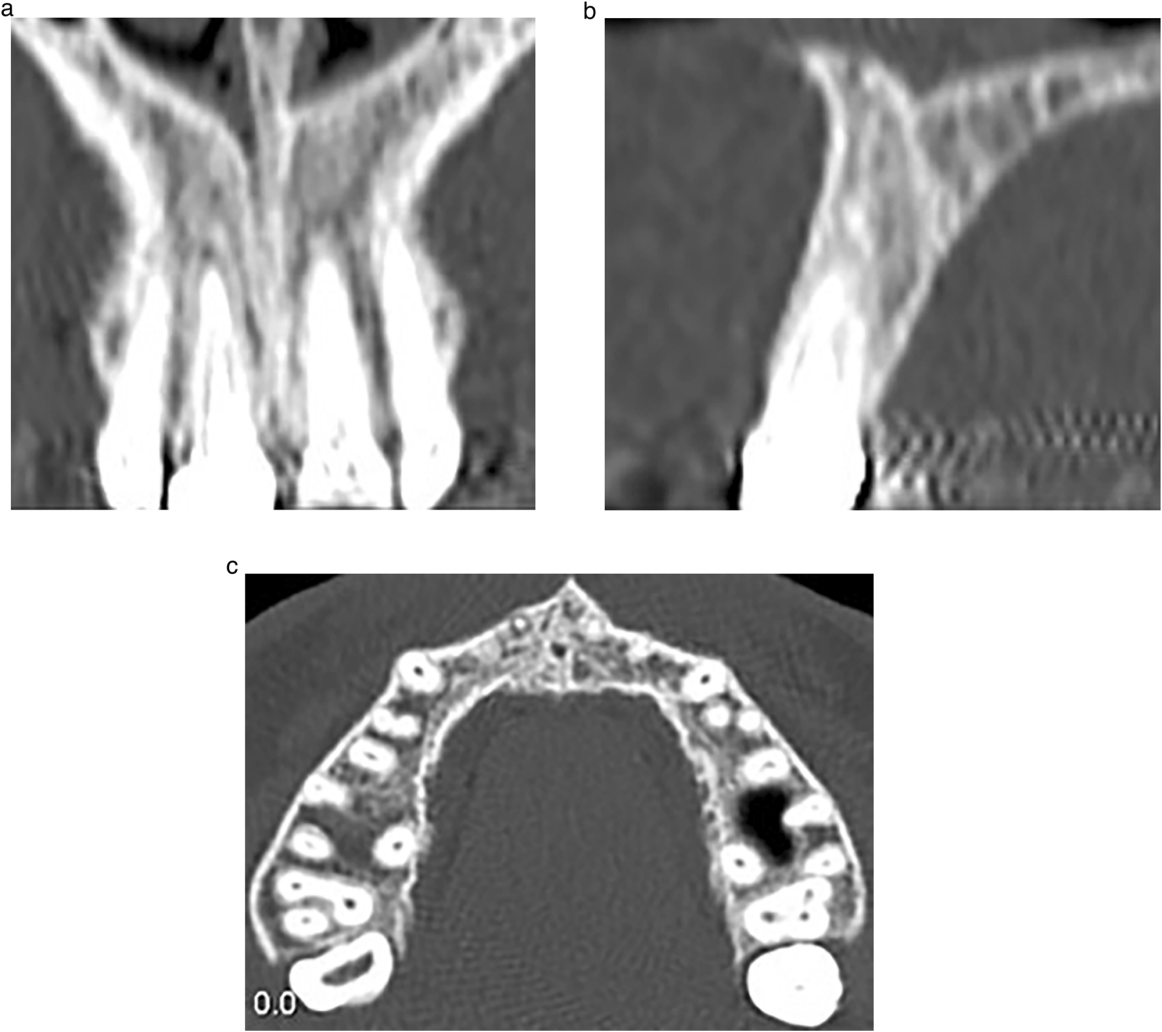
The second radiographical follow- up views. CBCT image after 12 months of treatment; a: Coronal view, b: Sagittal view, and c: Axial view.
